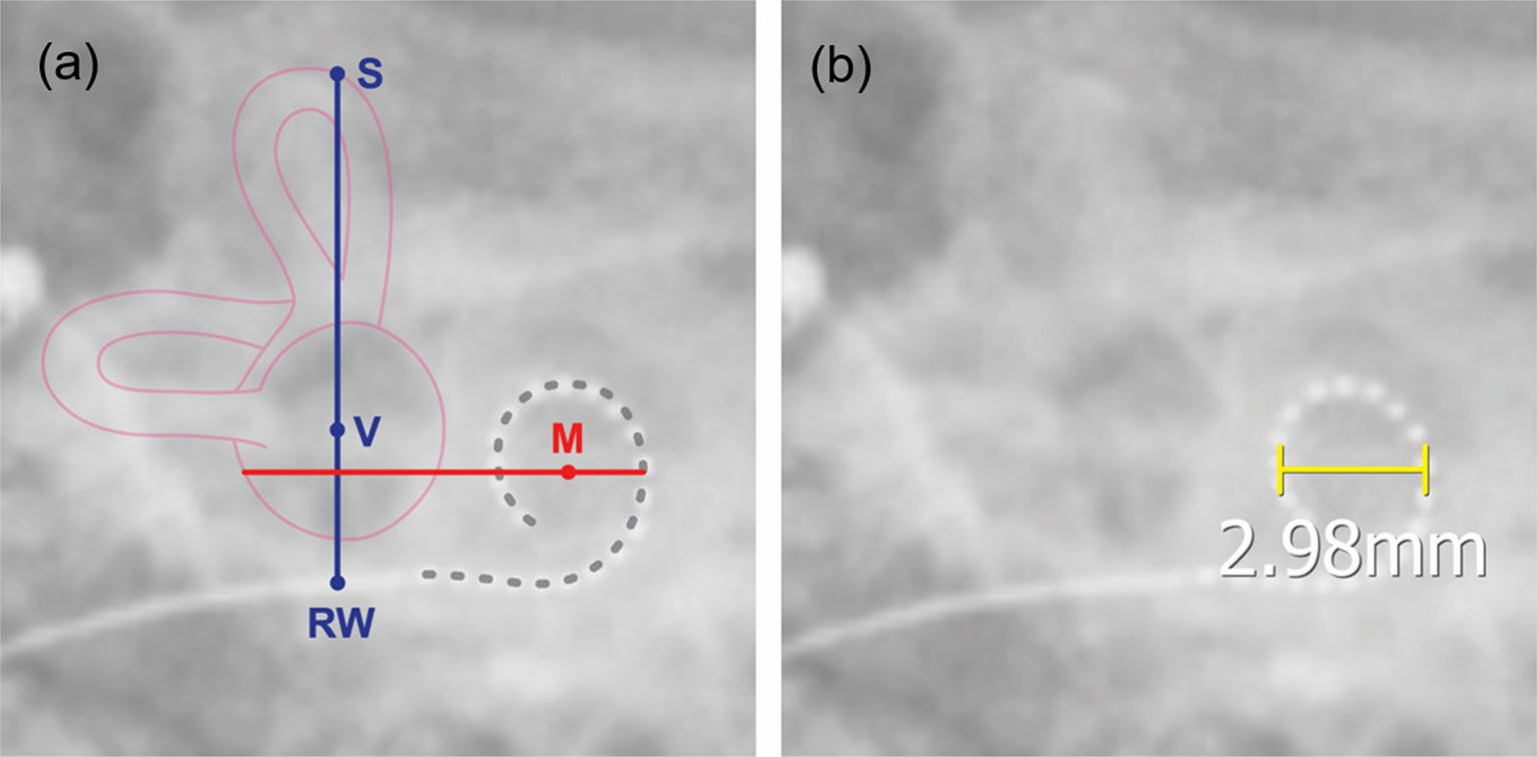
Landmark -based measurement of a spiral diameter on the postinsertion radiography. (a) The spiral diameter of the spiral configuration of the electrode array was measured on a horizontal line (red line) across the modiolus (M). This line is positioned vertically based on a reference line (blue line) connecting the apex of the superior semicircular canal (S) to the vestibule (V) and the round window (RW). (b) A representative X-ray-based spiral diameter on an unmarked radiograph of a right cochlea.
